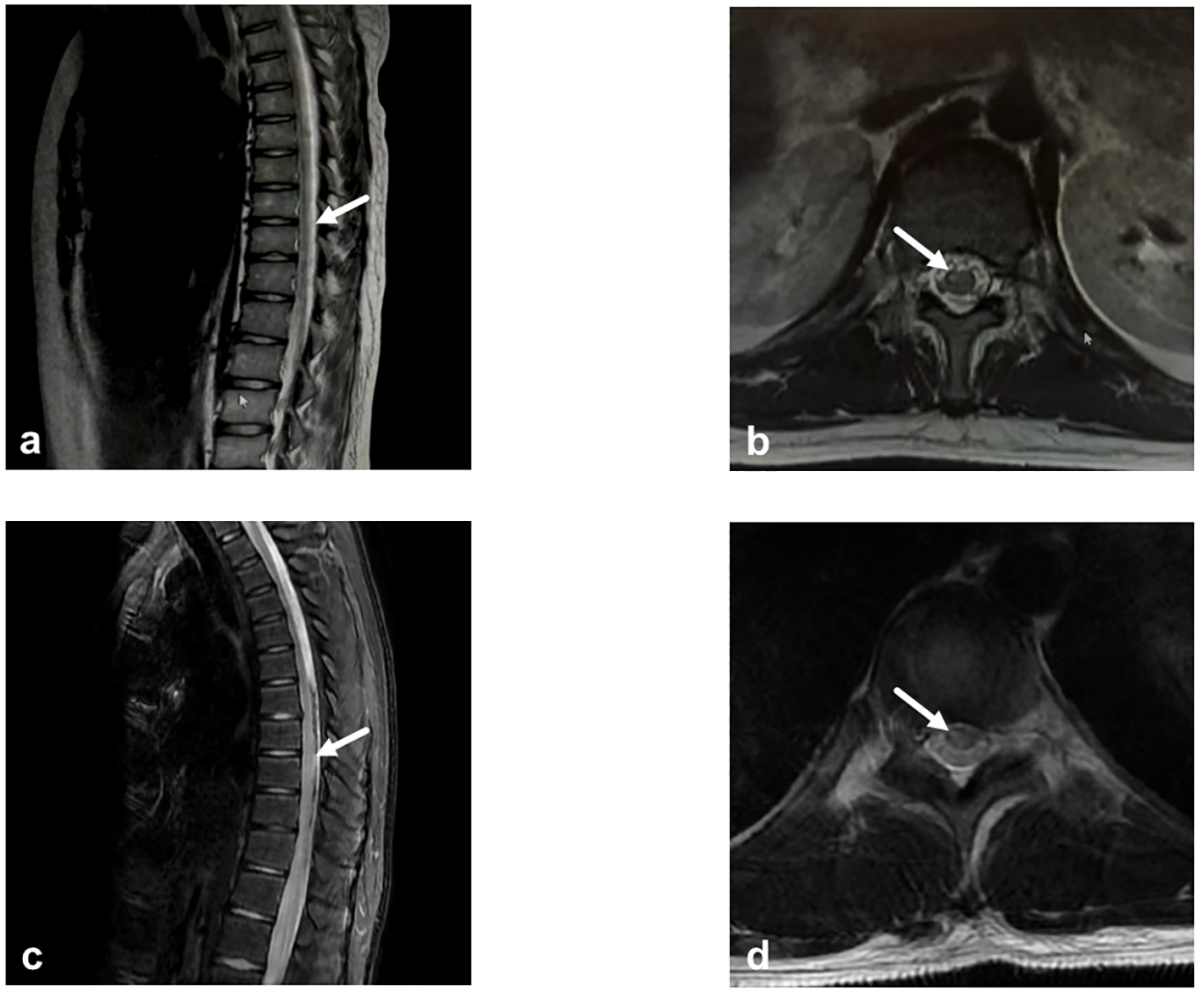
Imaging changes before and after ofatumumab treatment for case 1. (a) Sagittal T2-weighted thoracic spinal MRI. A patchy area of T2-weighted hyperintensity is observed within the spinal cord from T6 to L1, consistent with myelitis. There is a slight increase in epidural fat within the lumbar and sacral canal, resulting in compression of the thecal sac. (b) Axial T2-FLAIR thoracic spinal MRI. Reveals an abnormal signal focus in the right side of the spinal cord. (c) Sagittal T2-weighted thoracic spinal MRI. Demonstrates post-myelitis treatment changes. Following OFA treatment, thoracic spinal cord imaging shows a significant reduction in the extent of diffuse signal intensity compared to previous studies. (d) Axial T2-FLAIR thoracic spinal MRI. The previously noted abnormal signal in the right spinal cord has nearly resolved. OFA, Ofatumumab.
